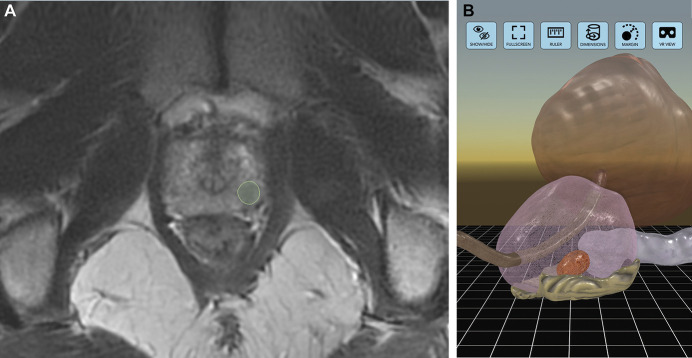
A*, MRI image of prostate showing lesion (outlined). *B*, 3D model of prostate showing anatomical structures, lesions and color-coded segments from prostate biopsy.
